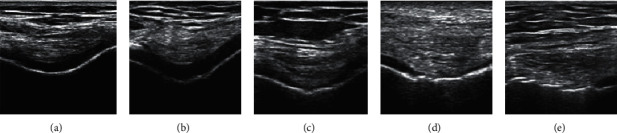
The ultrasound imaging manifestations of different stages in the experimental group. (a) Normal. (b) Stage I. (c) Stage II. (d) Stage III. (e) Stage IV.
